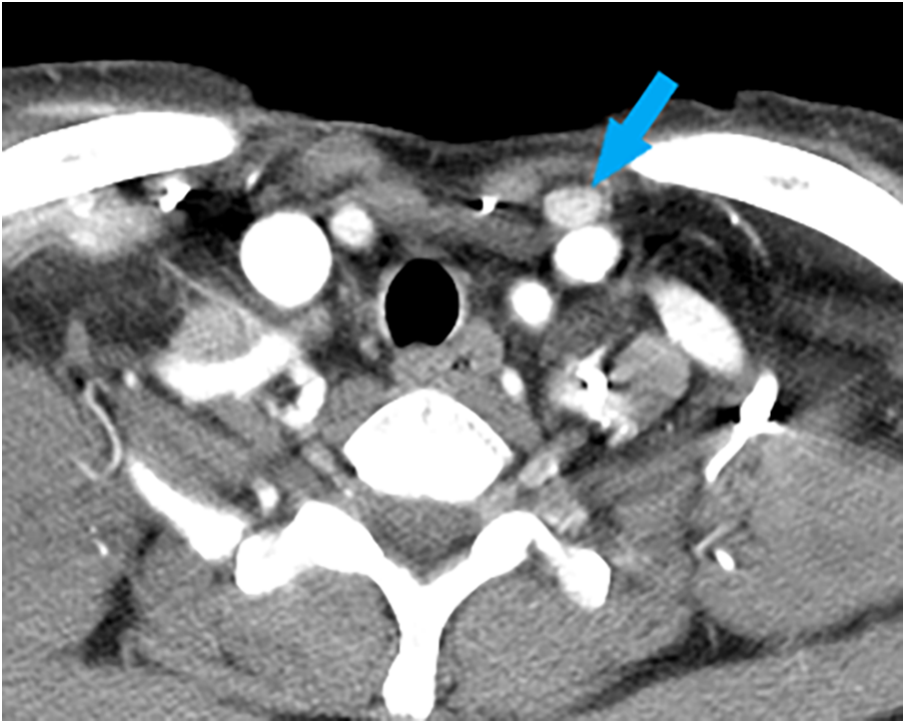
A computed tomography image of a metastatic lymph node (blue arrow), measuring 1.2 cm × 0.8 cm in size, at the left suprasternal space (SSLN).
